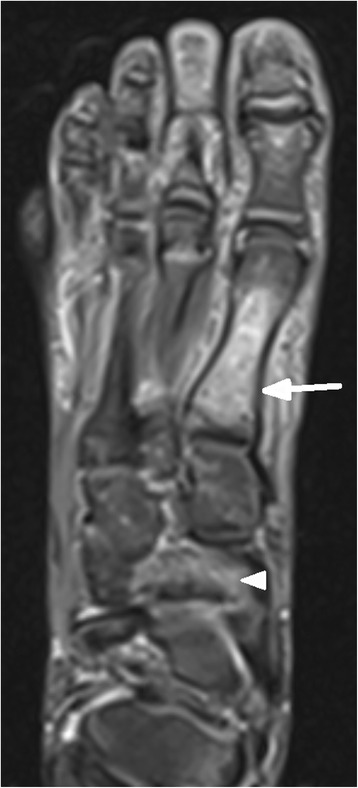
Axial STIR MR imaging shows marrow oedema of navicular (*arrow ahead*) and metatarsal I (*arrow*)
